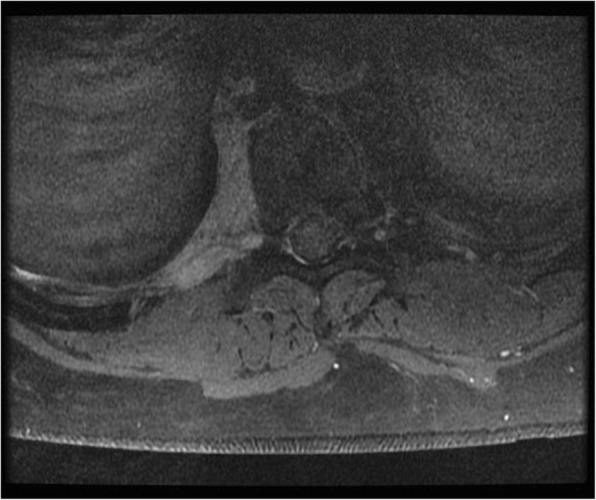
Transverse magnetic resonance imaging of the thoracic spine. T1-weighted image with gadolinium enhancement displaying abnormal paravertebral soft tissue thickening and enhancement of the right nerve root entering the neural foramina
